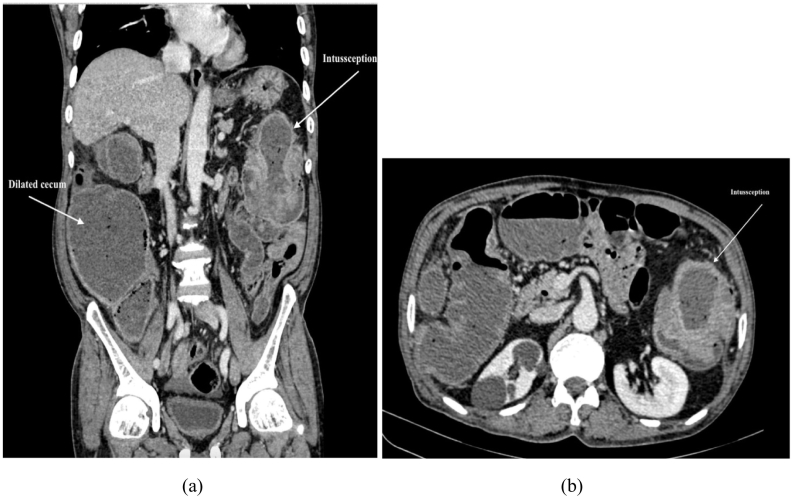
CT abdomen showing dilated caecum and telescoping of bowel segment into the descending colon.
